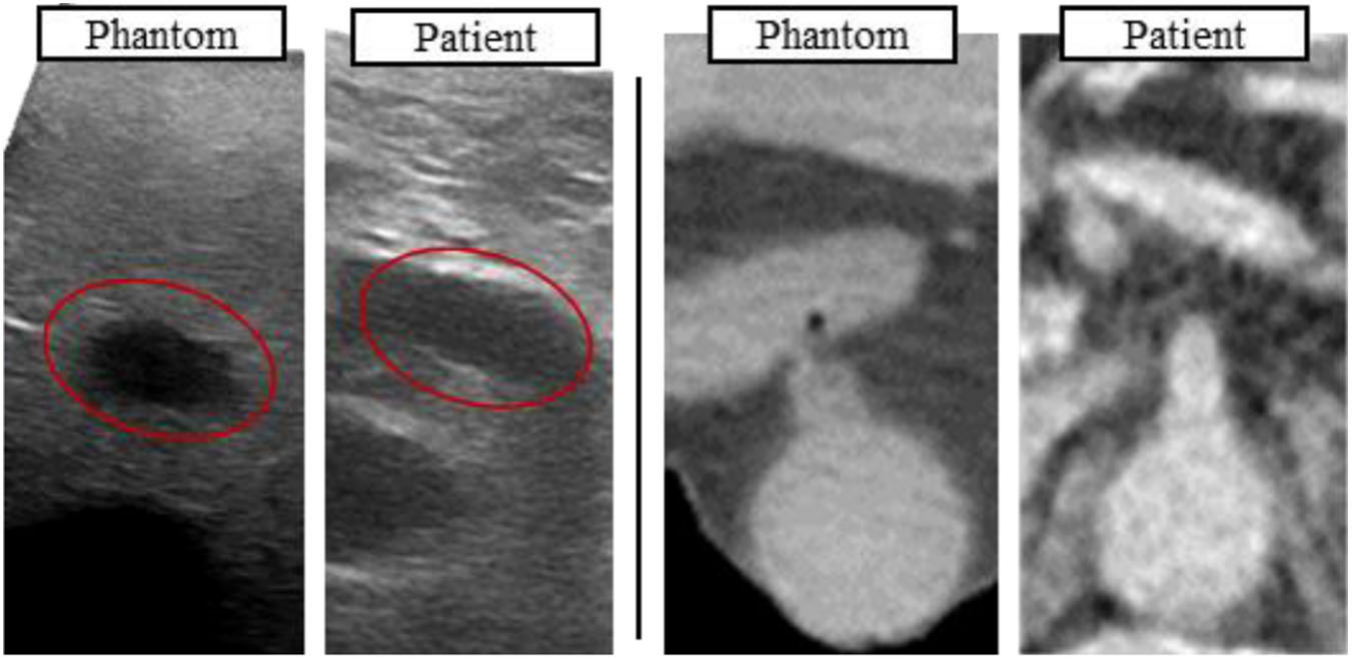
Comparison of imaging properties: (left) US image of hepatic artery (red outline) with patient US image acquired during open pancreas surgery; (right) cropped CT image of Aorta at SMA branch (patient CT scanned in PV phase).
